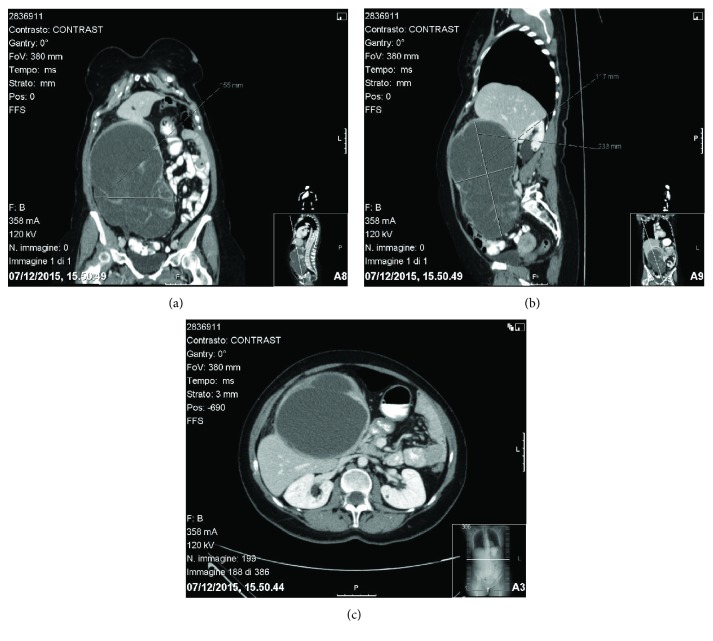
(a, b, c) A huge, partially cystic mass with heterogeneous contrast enhancement is seen in the right abdominal quadrant, adjacent to the liver (CT scan).
